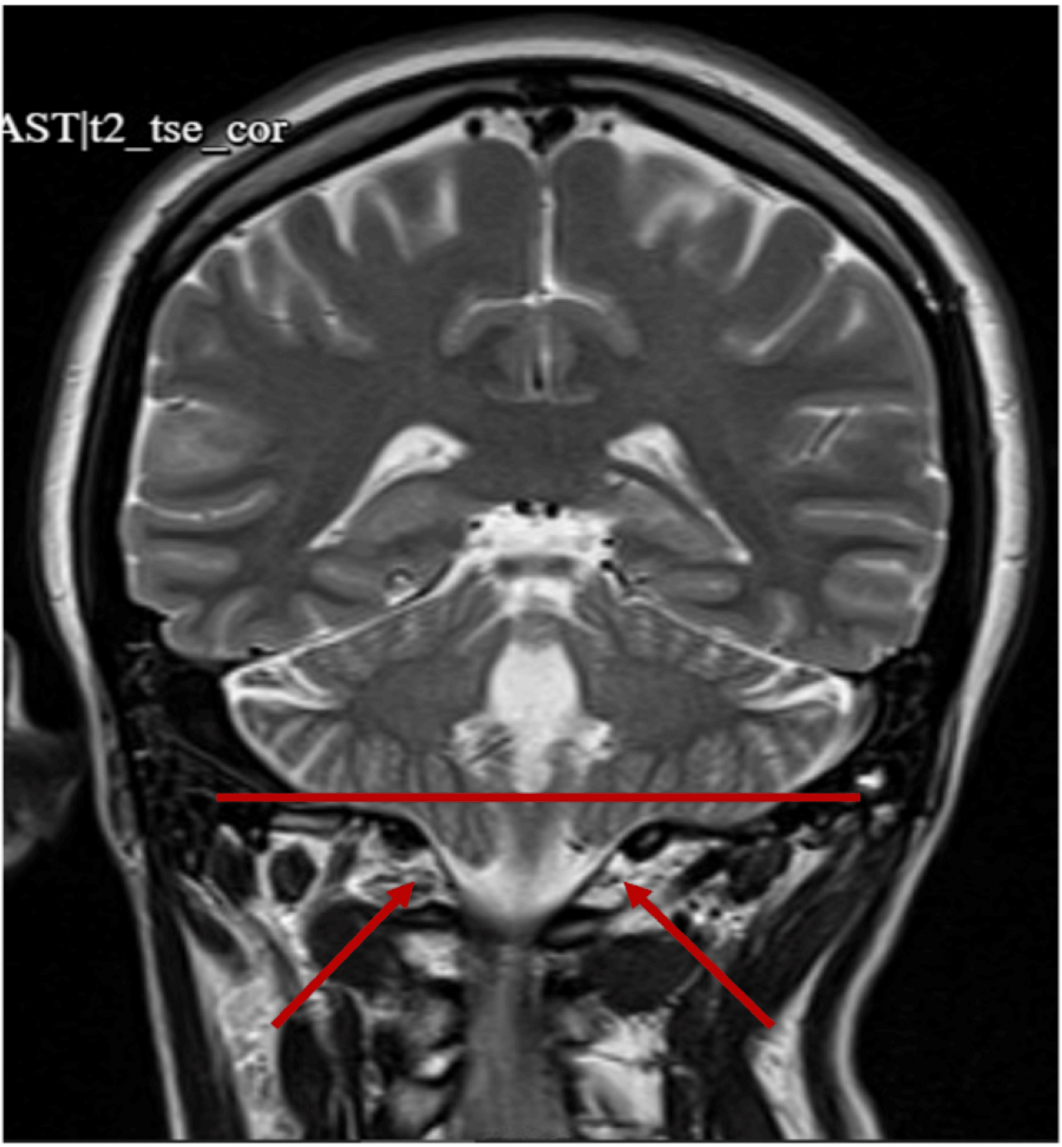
MRI of the brain The red arrows point out incidental finding of a low-lying right cerebellar tonsil extending 3 mm below the foramen magnum and mild paranasal sinus disease. MRI, magnetic resonance imaging.
